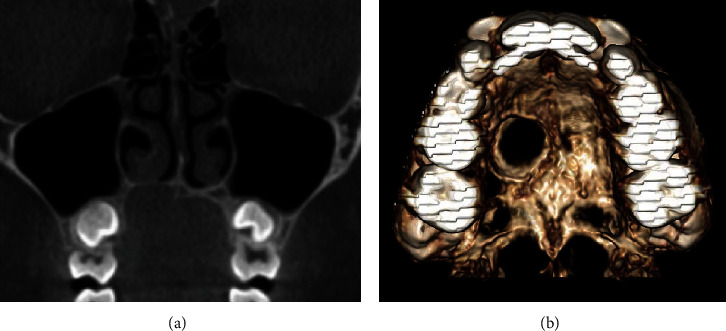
Cropped cone beam CT images of the maxilla. (a) Coronal image and (b) 3D reconstruction, showing concavity of the right hard palate, thinning, and superior displacement of floor of nasal cavity.
